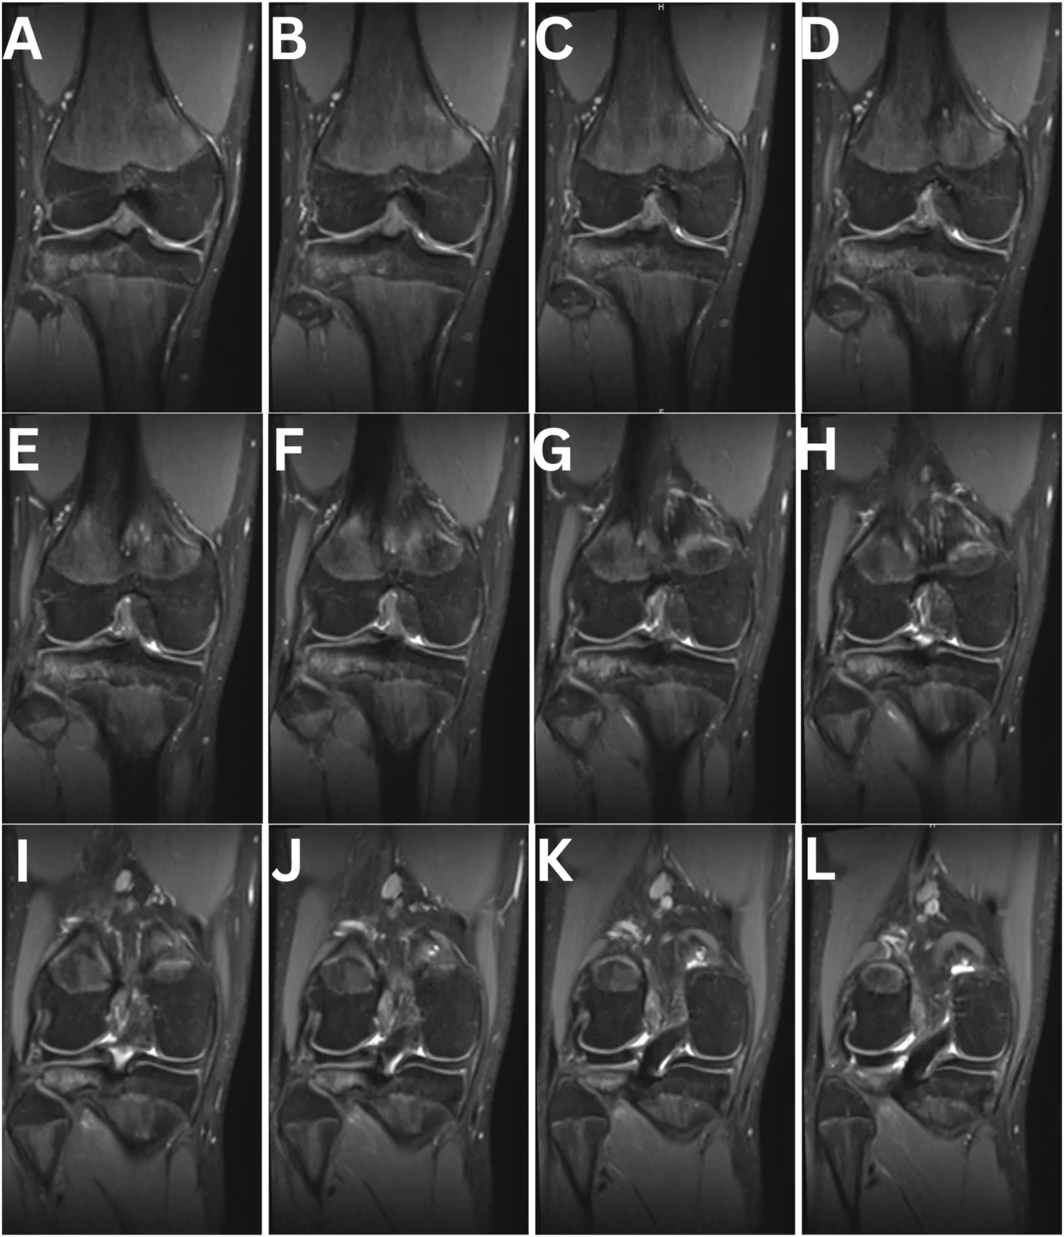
Initial coronal MRI. Coronal fat‐suppressed proton density–weighted images (A–L, anterior to posterior) show absence of the normal taut ACL band on mid‐notch slices (E–J), with heterogeneous tissue and fluid signal in the intercondylar notch consistent with a torn ACL remnant. Bone‐marrow edema is present in the lateral femoral condyle and lateral/posterolateral tibial plateau (A–H). Joint effusion is present. The PCL and collateral ligaments remain intact, with no displaced fracture or Segond‐type avulsion.
